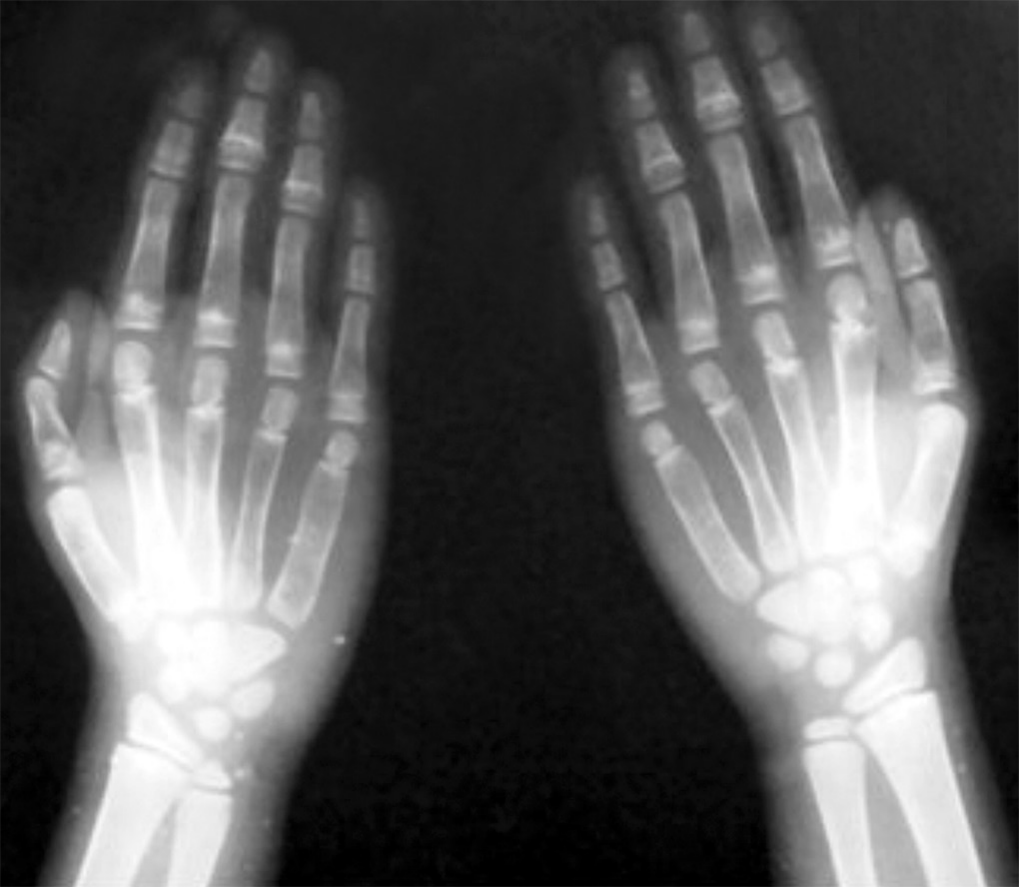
Hand wrist radiograph showing hypoplastic phalanges
